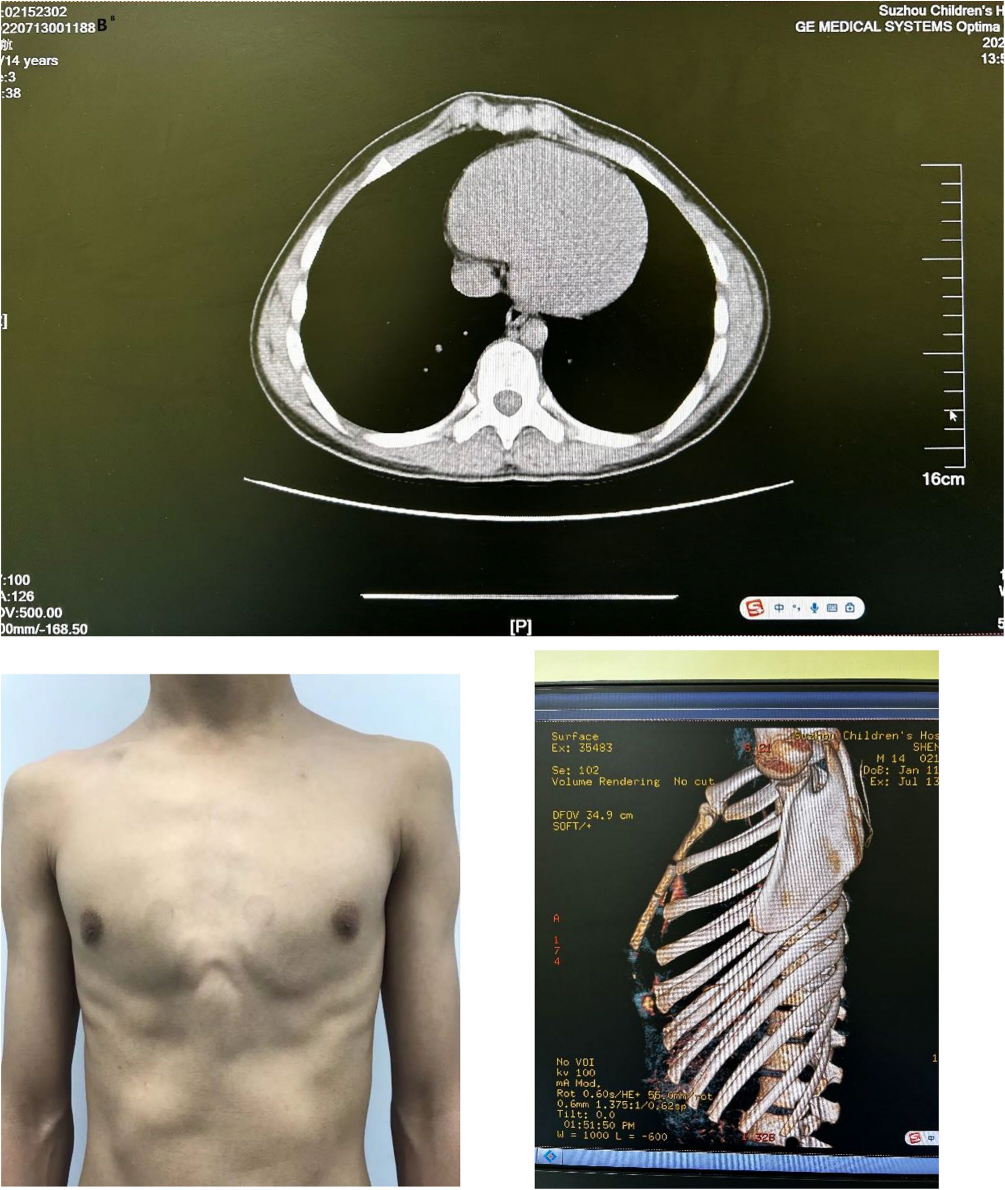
Chest appearance and CT scan of symmetrical PC
